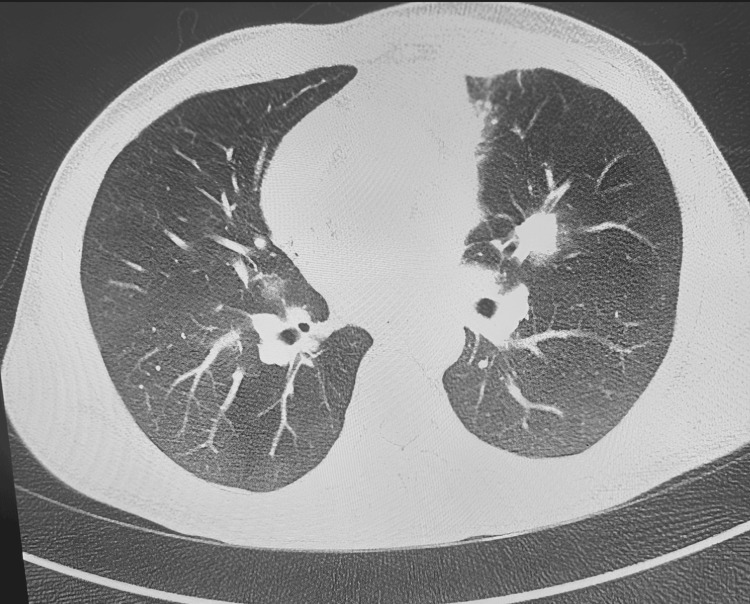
CT pulmonary angiography showing bilateral pulmonary soft tissue masses with no evidence of pulmonary embolism
